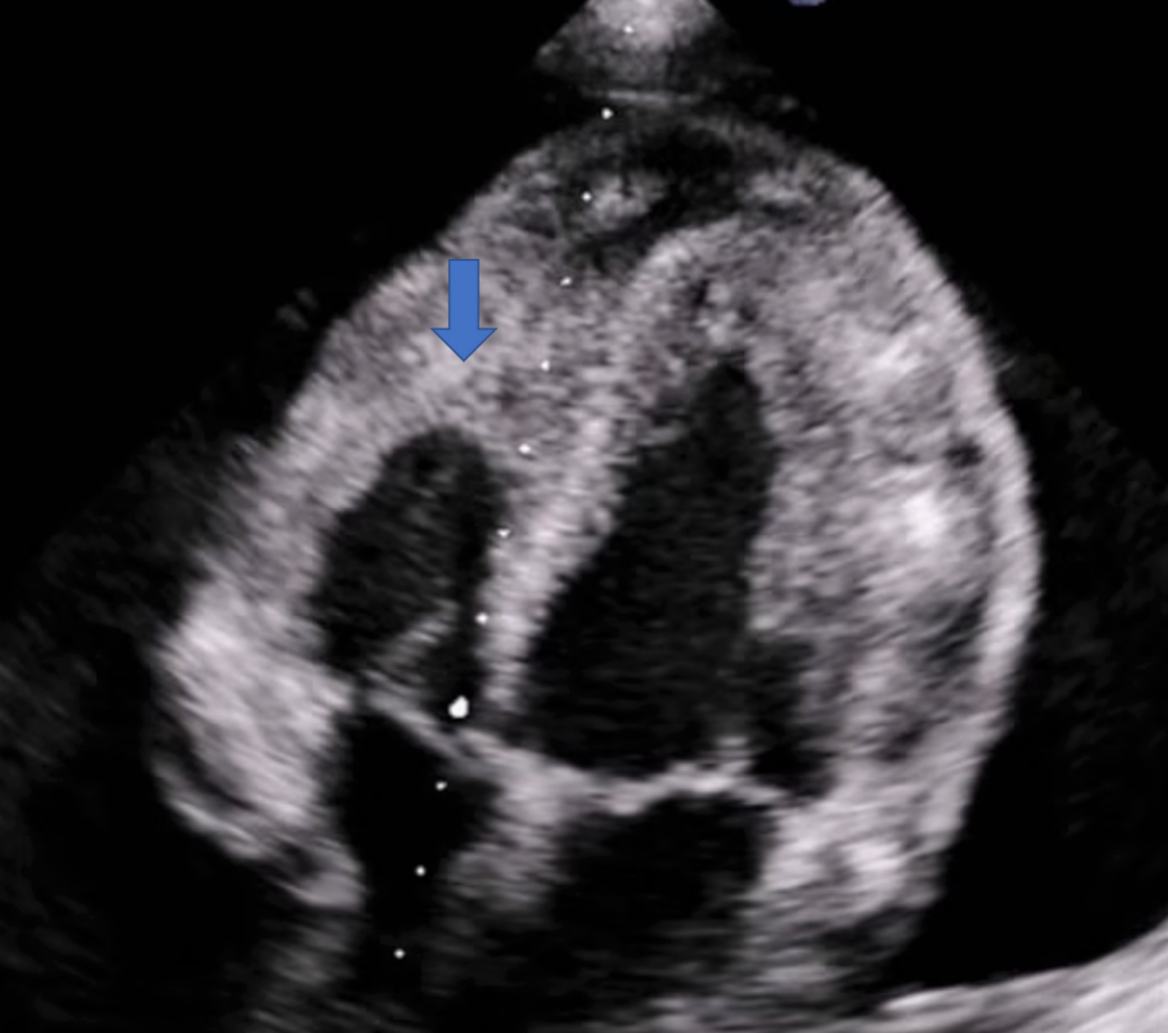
Echocardiogram showing the diastolic collapse of the right ventricle as indicated by the arrow in the figure
